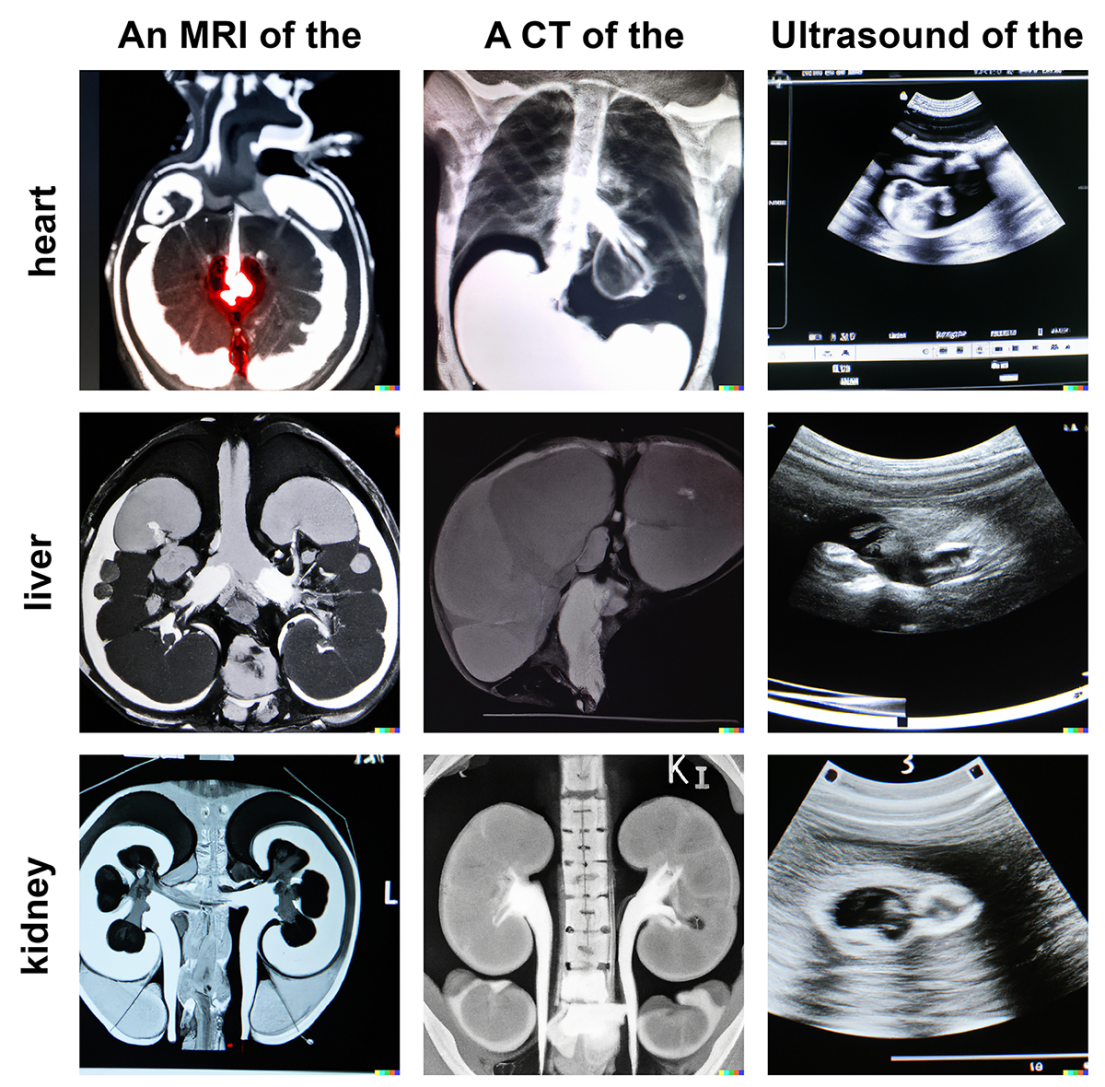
Examples of text-to-image–generated anatomical structures in CT, MRI, and ultrasound images created with DALL-E 2. CT: computed tomography; MRI: magnetic resonance imaging.
